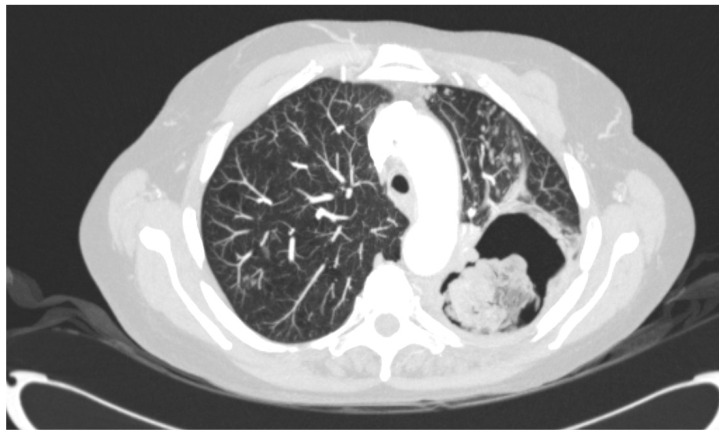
Chest computed tomography image showing a large pulmonary cavity in the left upper lobe with a mass lying in the cavity.
